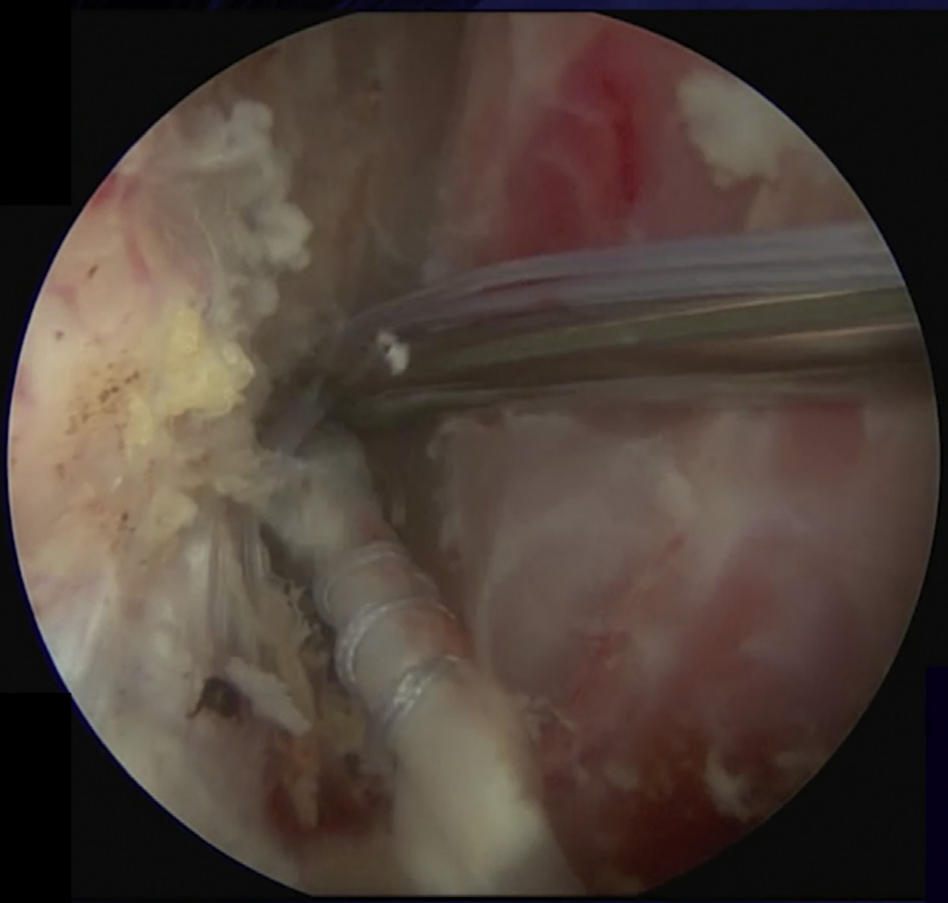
Subcoracoid view of a left shoulder from an ASL viewing portal. The LHB is pushed between the SSc and the CT. (ASL, anterosuperolateral; CT, conjoint tendon; LHB, long head of the biceps; SSc, subscapularis.)
